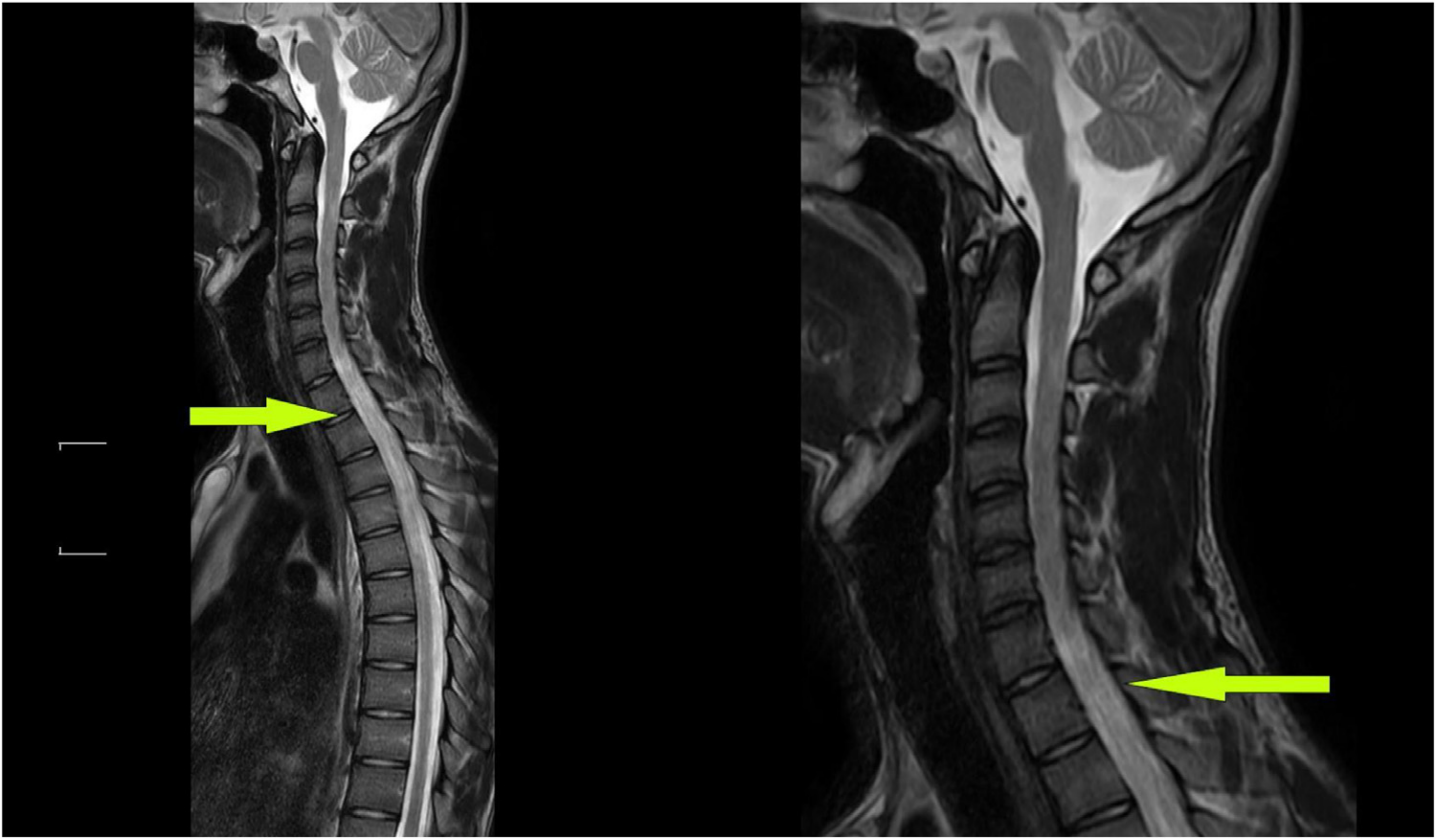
T2 sagittal FAT SAT MRI images showed intramedullary long segment hyperintense signals extending from C3 to T3 level of spinal cord.
